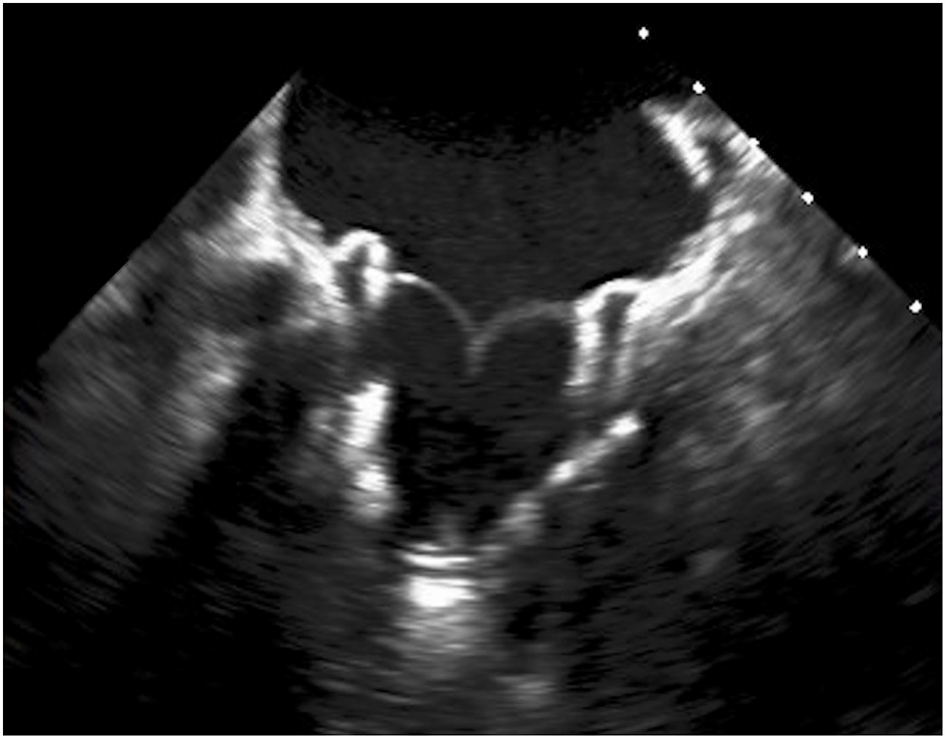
Transesophageal echocardiography showing Tendyne^TM^ valve (Abbott) after deployment.
